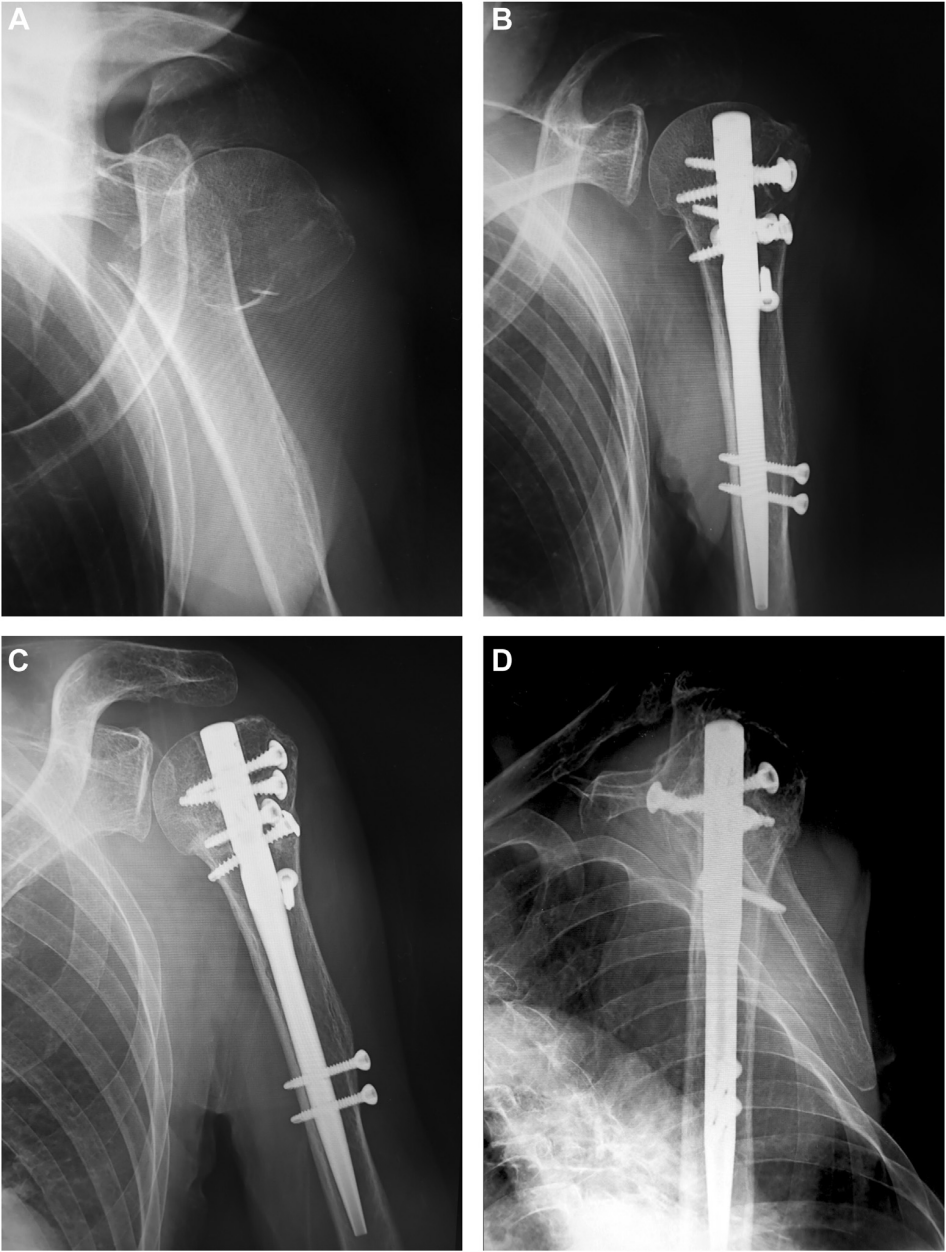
Left* humeral surgical neck fracture of an 87-year-old woman. (A) Anteroposterior radiograph at the time of injury. The distal fragment shows medial translation of 65.4%, and the preoperative neck-shaft angle is 168°. (B) Anteroposterior radiograph 2 weeks after osteosynthesis. Intramedullary nailing with blocking screw augmentation reduces medial translation, and the neck-shaft angle is 142°. (C) Anteroposterior radiograph at the final follow-up 12 months after osteosynthesis. Bone union is achieved without any correction loss. (D) Scapular-Y radiograph at the final follow-up. Posterior angulated deformity does not remain.
